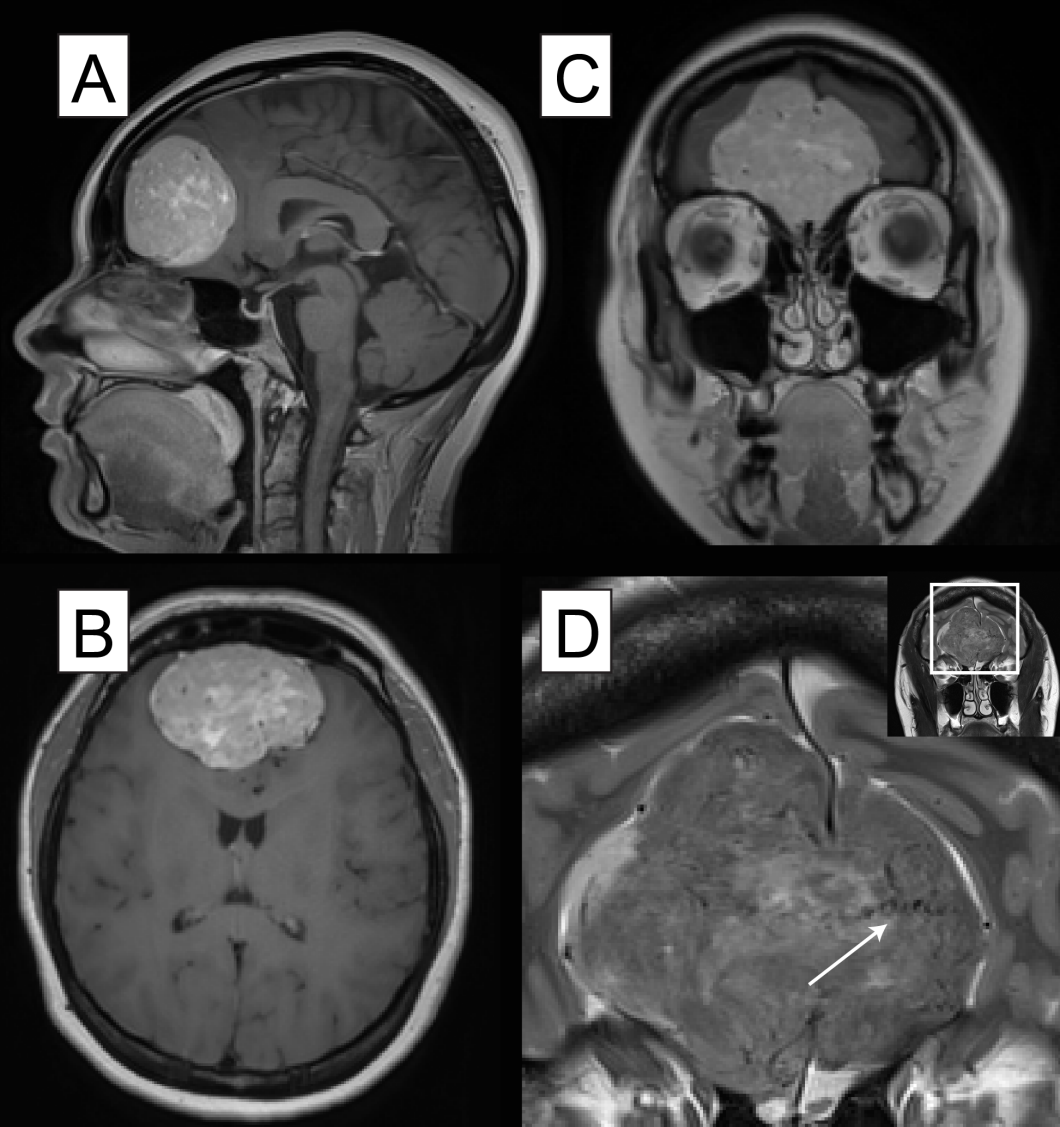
The preoperative magnetic resonance imaging (MRI). The magnetic resonance imaging (MRI) with contrast (A) sagittal (B) axial, and (C) coronal views depict a large contrast-enhancing mass arising from the olfactory groove, D: the MRI T2 weighted image demonstrates corkscrew type flow voids (white arrow), which are consistent with a highly vascular neoplasm.
